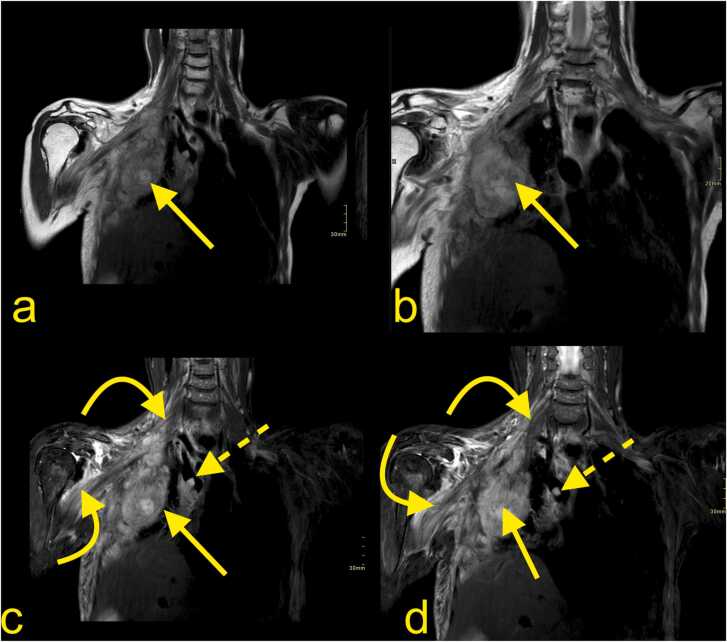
A 62-year-old patient with generalized HER2-positive breast cancer since 2001. There were manifestations in the lungs, lymph nodes, and skeleton (straight arrow). The patient's biggest problem was swelling, aches, poor motor function, and sensation around the right scapula, in the shoulder, and arm. MRI of the BP with (a, b) T2-weighted DIXON TSE and (c, d) T2-weighted DIXON TSE with fat suppression showed an extensive tumor mass (straight arrow) originating from the thoracic wall and infiltration of the BP. Both the supra- and infraclavicular parts of the BP are swollen with increased signals (curved arrow). Vascular invasion is seen in the superior vena cava (straight dashed arrow).
